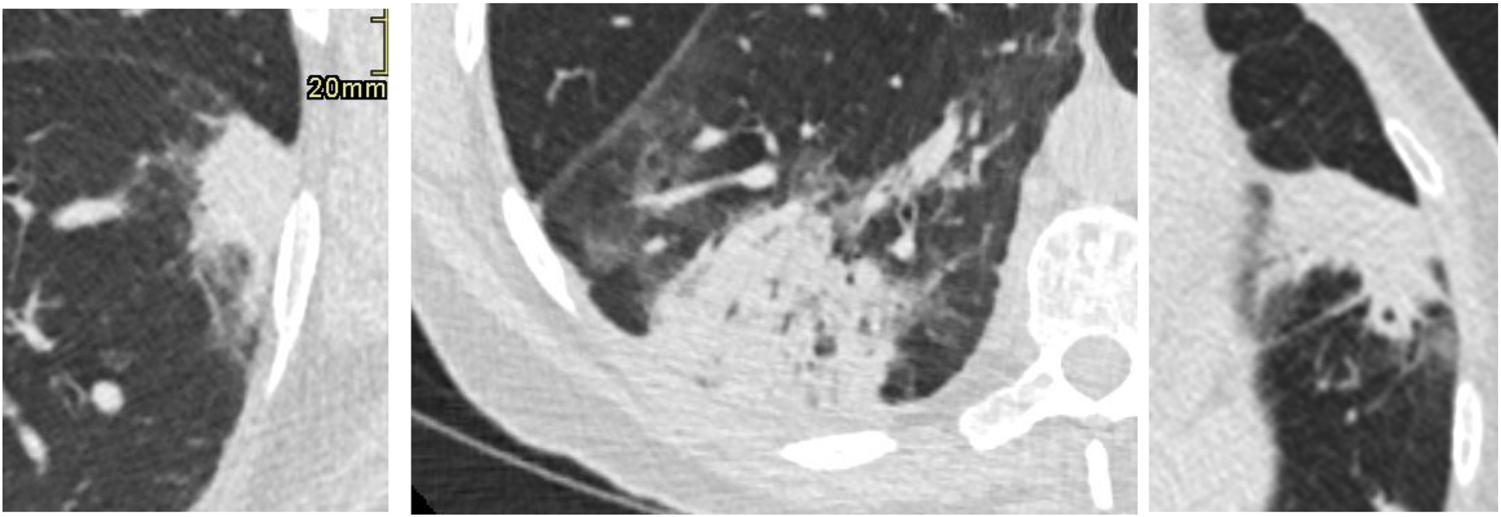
Examples of focal consolidation, for which 6-week follow-up CT was recommended
